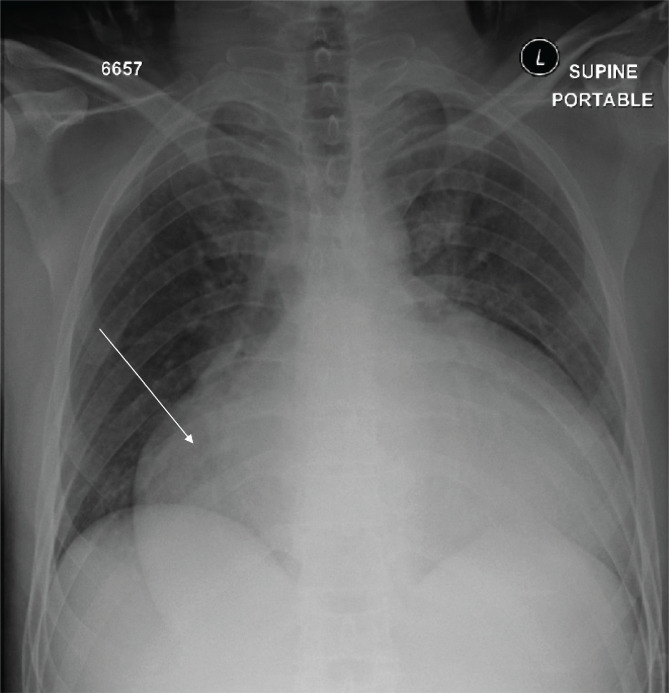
Chest X-ray; the arrow indicates the massive pericardial effusion.
